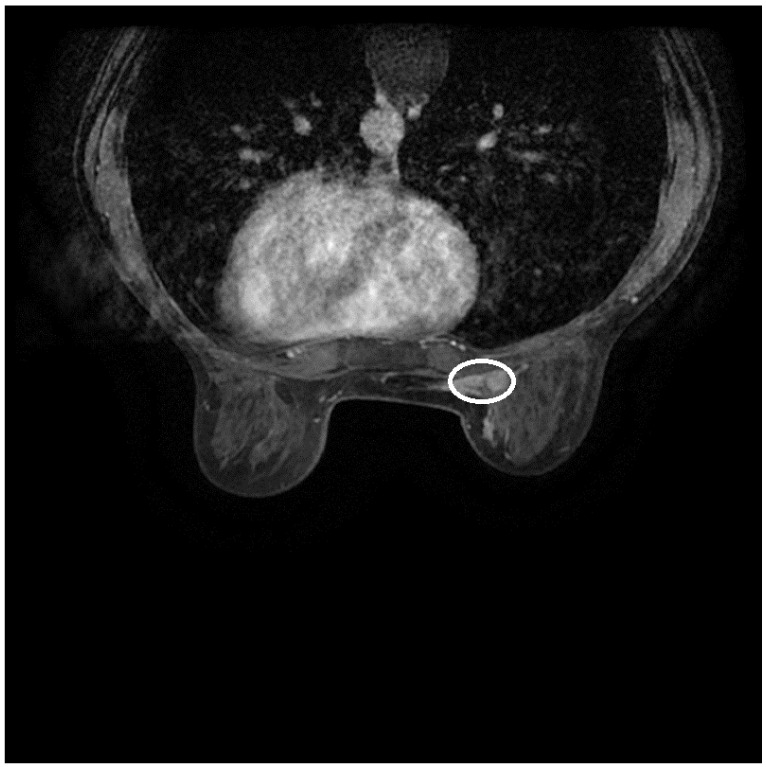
MRI of right breast tumor. Contrast uptake at the level of the right breast tumor.
